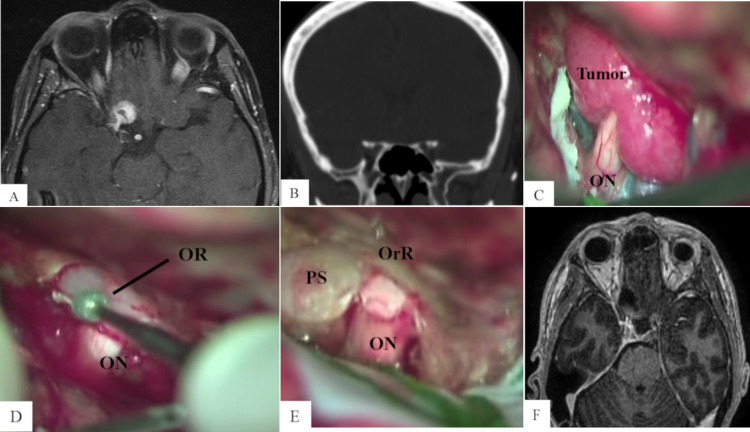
Tailored intradural AC (optic canal unroofing) (A) Preoperative contrast-enhanced axial T1-weighted MRI shows a small type III anterior clinoidal meningioma on the right side extending into the optic canal. (B) Preoperative coronal bone window CT scan does not show hyperostosis of the ACP. (C) Tumor involving the optic nerve and its attachment to the ACP. (D) The tumor was removed and the dura over the roof of the optic canal was cut, preparing for optic canal unroofing with a 2-mm high-speed diamond drill. (E) A small piece of the tumor was removed from the optic canal and gross total resection was achieved. (F) Postoperative contrast-enhanced axial T1-weighted MRI shows total tumor resection. ON, optic nerve; OR, optic roof; PS, planum sphenoidale; OrR, orbital roof; CT, clinoid tip
